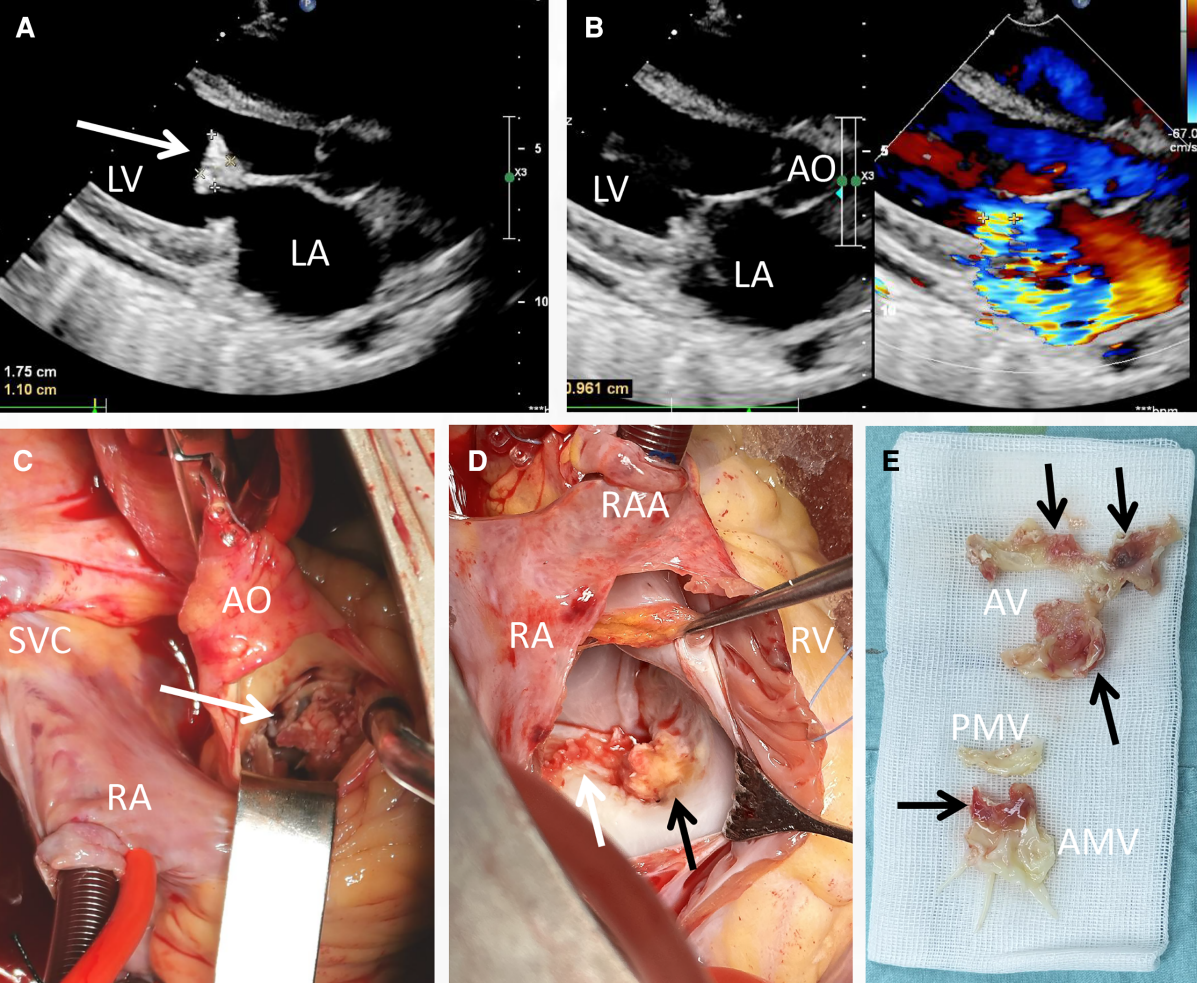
Transthoracic echocardiogram and intraoperative view of the vegetations. A vegetation sizing 1.75 cm × 1.10 cm (A, arrow), as well as severe mitral regurgitation (B) were detected pre-operatively; the yellowish vegetation (arrow) was observed intra-operatively with the infiltration of aortic valve (C), anterior mitral cusp (D) and mitral annulus (E).
